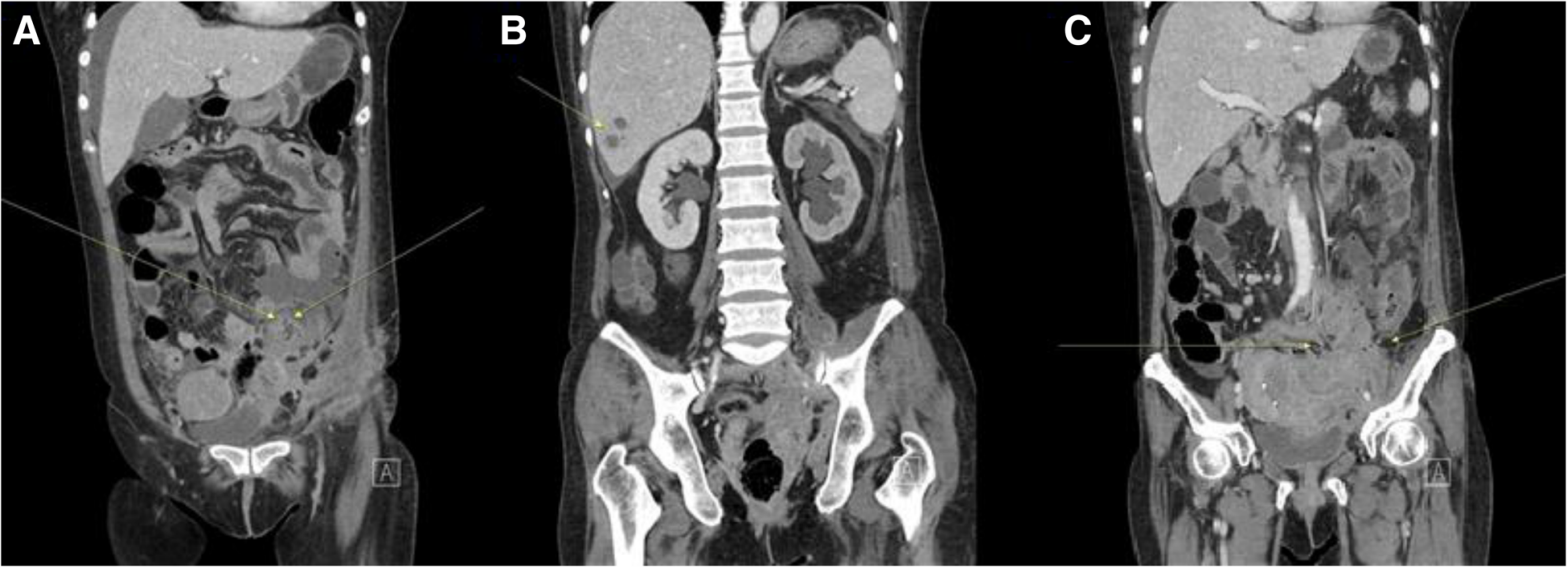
CT finding at the emergency room. a CT scan of the abdomen-pelvis revealed a microperforation (*arrow*) of the sigmoid colon and abscess in the left lower quadrant. b CT scan showed a hepatic lesion (*arrow*) and bilateral hydronephrosis. c There was a large infiltrating heterogenous hyperattenuating conglomerated mass invading the urinary bladder, left adnexa, sigmoid, left inguinal canal and left pelvic wall area (*arrow*)
